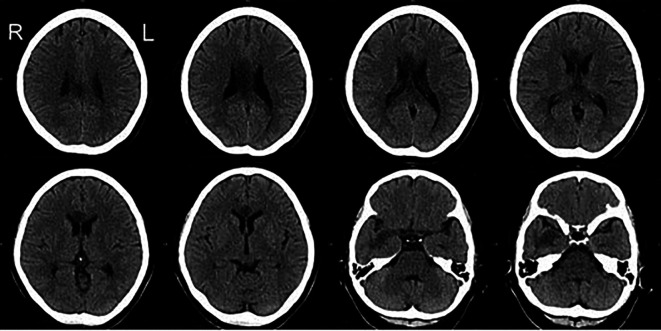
Axial view of the CT Brain. The CT images demonstrate hypodensity over the corpus callosum extending from the right genu to the left side of the splenium and the right temporal lobe. CT, computed tomography.
